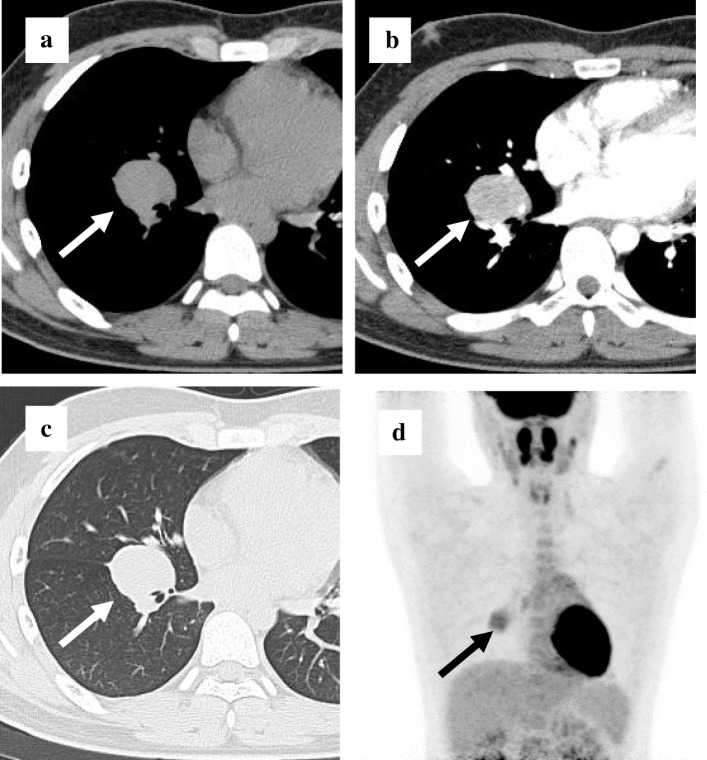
Radiological findings. a Non-enhanced chest CT of mediastinal condition showed a 35-mm mass in the right pulmonary hilum (arrow). b In enhanced chest CT of mediastinal condition, the tumor was strongly enhanced in the early phase (arrow). c In non-enhanced chest CT of lung field condition, abnormal findings were not found in the lung field. d FDG-PET scan showed accumulation only in the mass (arrow)
